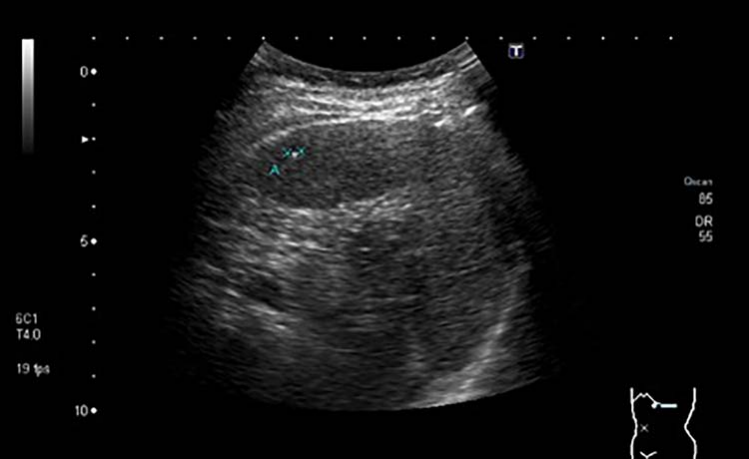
Ultrasound showing a hypoechoic nodule, vaguely heterogeneous, located in segment VII, subcapsular, and with ~22 mm in diameter.
